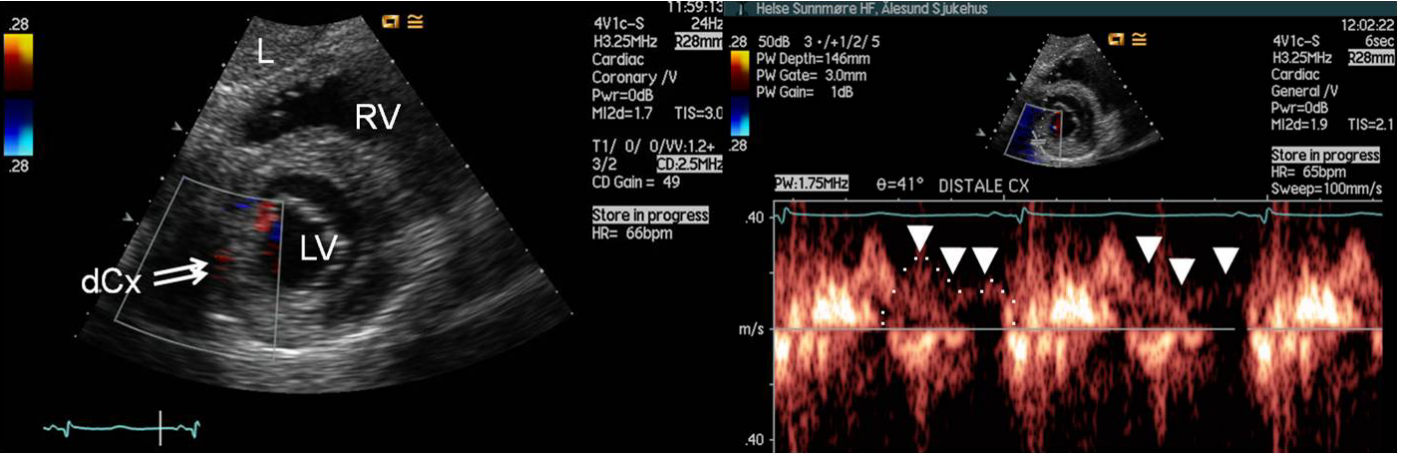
Example of antegrade coronary artery flow in the distal segment of Cx. The distal segment of the circumflex coronary artery (dCx) imaged by colour Doppler mapping and matching spectral Doppler tracings of blood flow (with arrowheads denoting diastolic flow waveform with one diastolic flow velocity waveform enveloped), imaged from modified subcostal short-axis view focusing on the inferior atrioventricular sulcus. L = liver; LV = left ventricle; RV = right ventricle.
